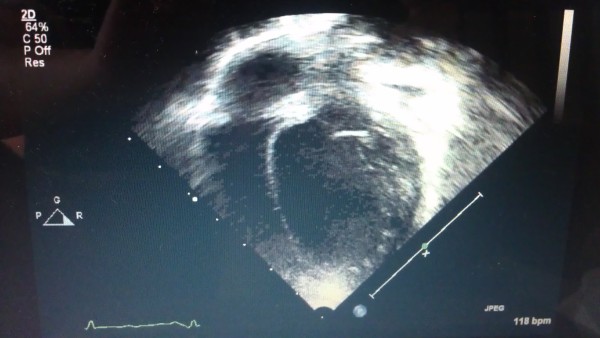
Echocardiography of a 15-month male infant with dilated myocardiopathy.
